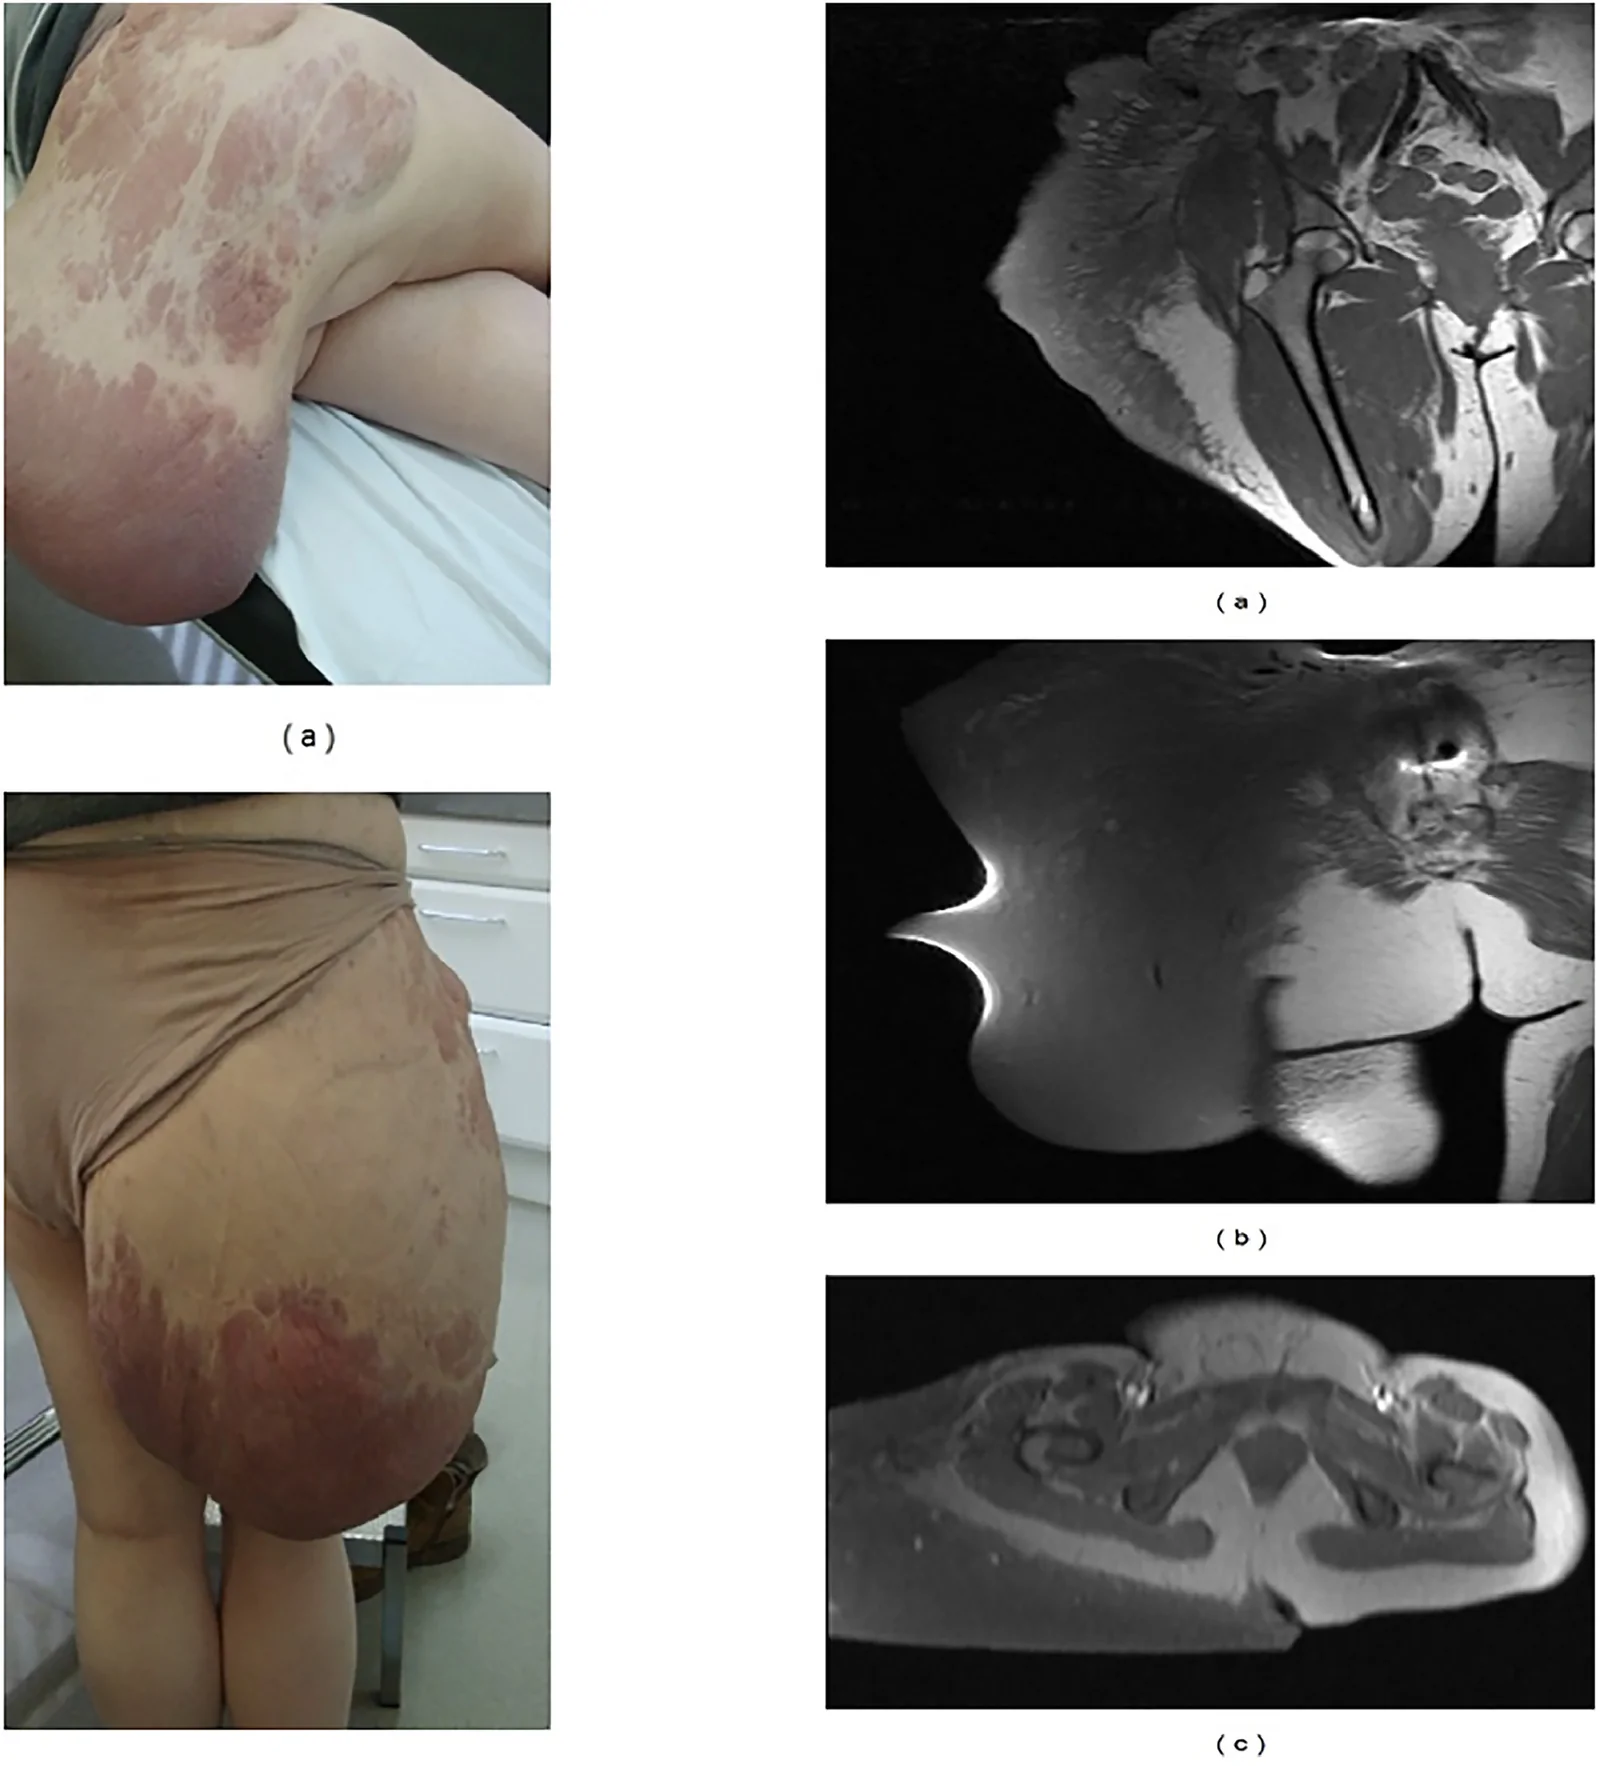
Preoperative images of a giant PN in the right buttock and lumbar region with associated MRI images. Note in the MRI images that there is a flow void, which is lack of image definition in the area of the PN due to the high flow vascularity within the tumour. Reproduced with permission from Vélez et al. 2013, Case Reports in Neurological Medicine, licensed under CC BY 4.0 (https://creativecommons.org/licenses/by/4.0/).
